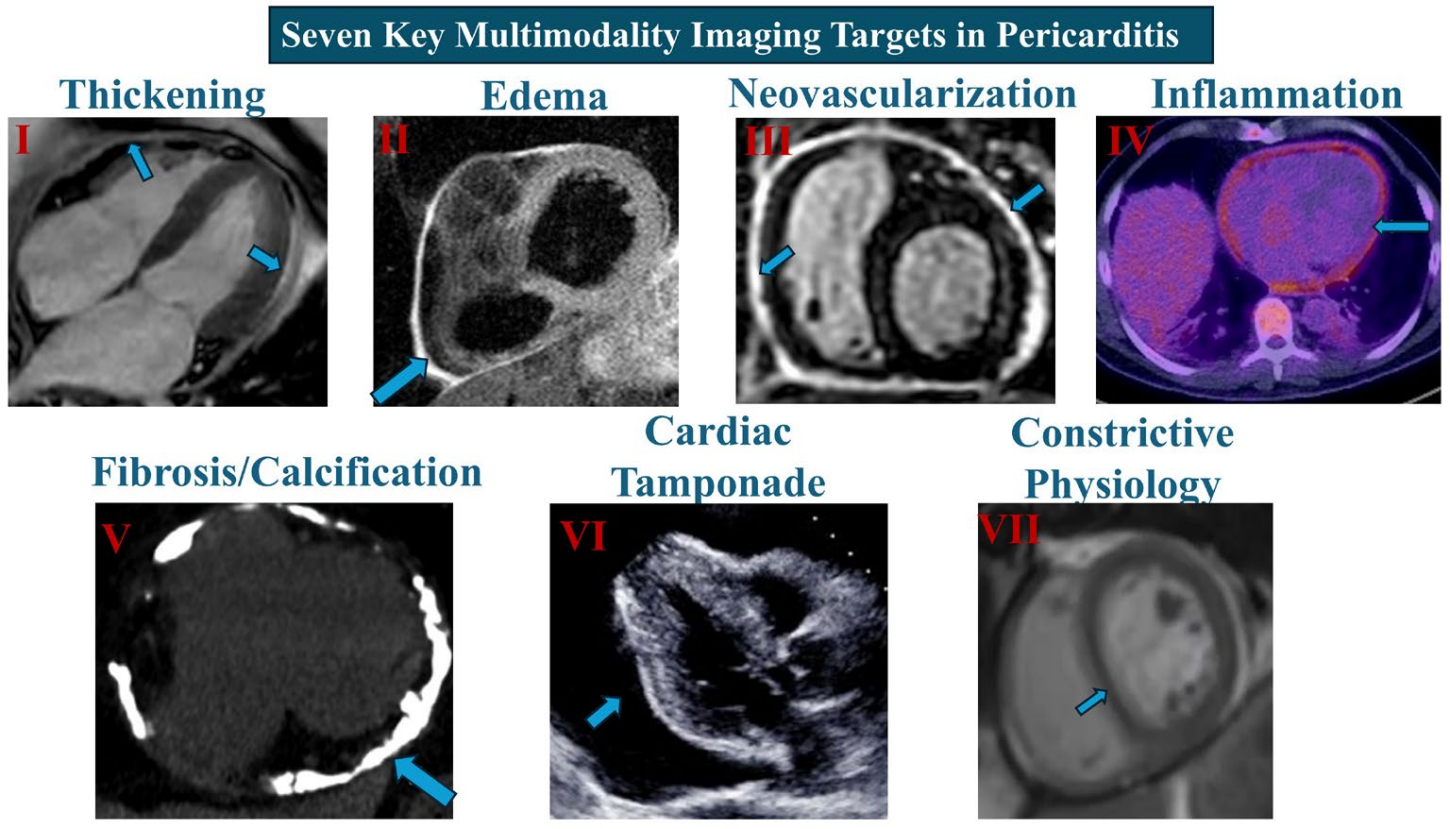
It depicts the 7 key imaging targets (roman numerals) that should be evaluated in patients presenting with pericarditis, with examples from multimodality imaging: I. Thickening: CMR cine‐4 chamber showing significantly thickened pericardium. II. Edema: Increased pericardial signal intensity on T2‐STIR weighted CMR imaging. III. Neovascularization: Circumferential LGE which is a surrogate for pericardial neovascularization and inflammation injury. IV. Inflammation: FDG‐PET CT showing diffuse circumferential pericardial uptake representing edematous and inflamed pericardium. TTE apical 4‐chamber view showing circumferential pericardial effusion. V. Fibrosis and Calcification: Cardiac CT showing extensive and almost circumferential pericardial calcifications. VI. Cardiac Tamponade Physiology (CTP): TTE parasternal long‐axis view showing large circumferential pericardial effusion. This patient had cardiac tamponade with evidence of right‐sided chamber diastolic collapse (not highlighted in this image). VII. Constrictive Physiology (CP): Real‐time free‐breathing sequence on CMR showing septal flattening as a sign of respirophasic septal shift indicating constrictive physiology.
